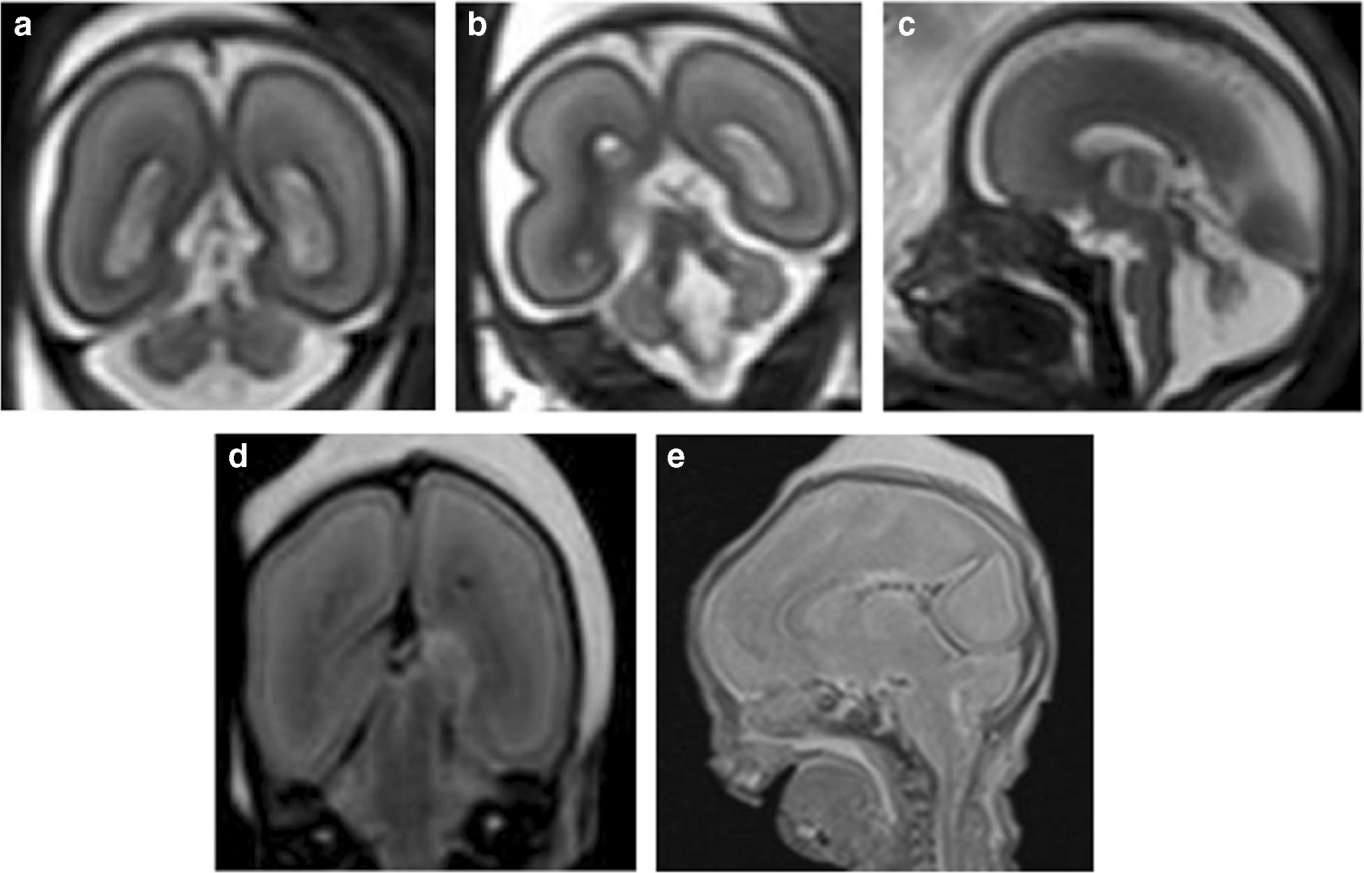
Abnormal cerebellar features in a 23-week gestation fetus. a, b Coronal T2-weighted iuMR images reveal features suggestive of an absent cerebellar vermis. The ‘buttocks sign’ is show in image a, and in image b, the ‘molar tooth appearance’ of elongated superior cerebellar peduncles is featured. c Sagittal T2-weighted iuMR image depicts the characteristic ‘figure of 7’ appearance of the elongated superior cerebellar peduncles and small cerebellum. d, e Coronal and sagittal T2-weighted PMMR images do not demonstrate the cerebellar abnormalities
